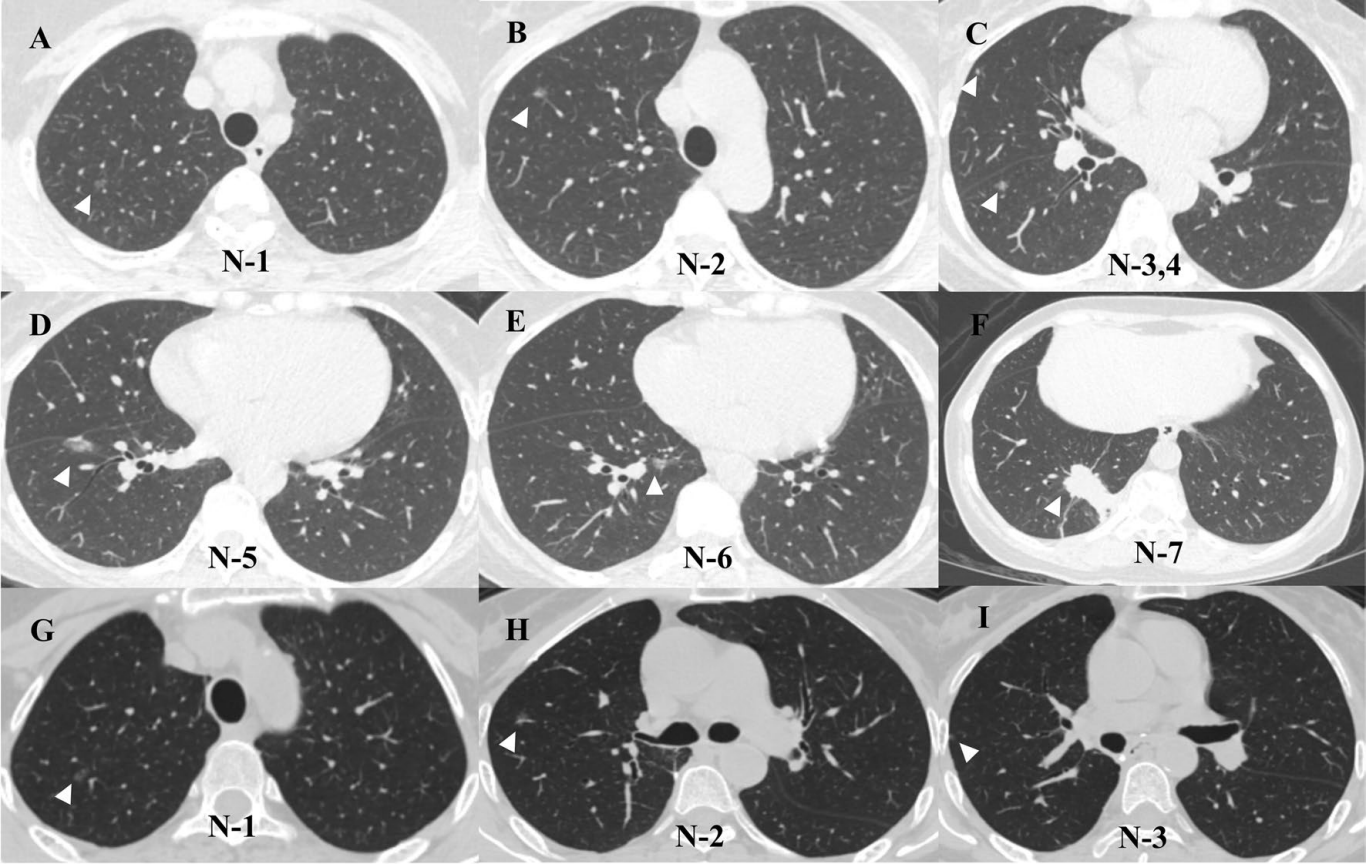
Chest CT scan finding of the patient. A–F the right upper lobe shows two 0.6 cm GGOs in S1 (A, B). the right middle lobe shows a 0.3 cm GGO in S5 (C). the right lower lobe shows an 0.8 cm GGO in S6 (C), a 1.6 cm GGO in S7 (D), a 1.3 cm partial solid nodule in S8 (E), and a 2.6 cm solid nodule in S10 (F). G–I Chest CT scan shows the remaining lesions' after thirty-six months of follow-up
